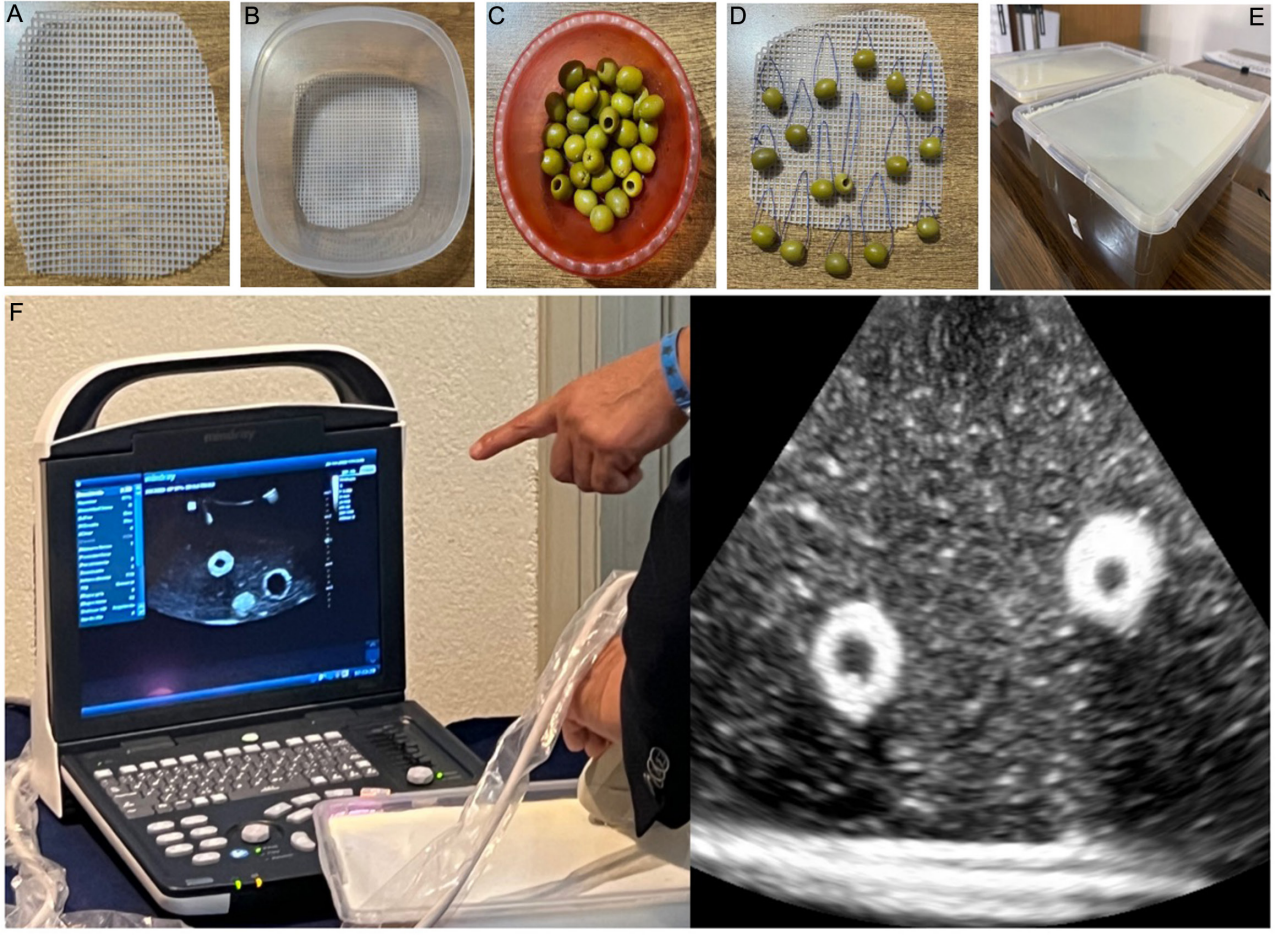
Model building. (A) Plastic mesh suited for the area of the inner base of the container. (B) Adjust the size of the plastic mesh to fit the container base. (C) Pitted olives. (D) Thread the olive’s interior with a piece of string and knot it to the plastic mesh to prevent floating when gelatin is poured; each olive represents a target. (E) For dense gelatin concentration, use 160g gelatin (300 bloom) per liter of water. Boil, stir, and add 1 tablespoon Psyllium-Plantago. Pour into a container and cover olives at the base until 8 cm thick. When the mixture is firm, apply a thin layer of liquid latex to the surface. (f) Ultrasound image of the model to puncture.
